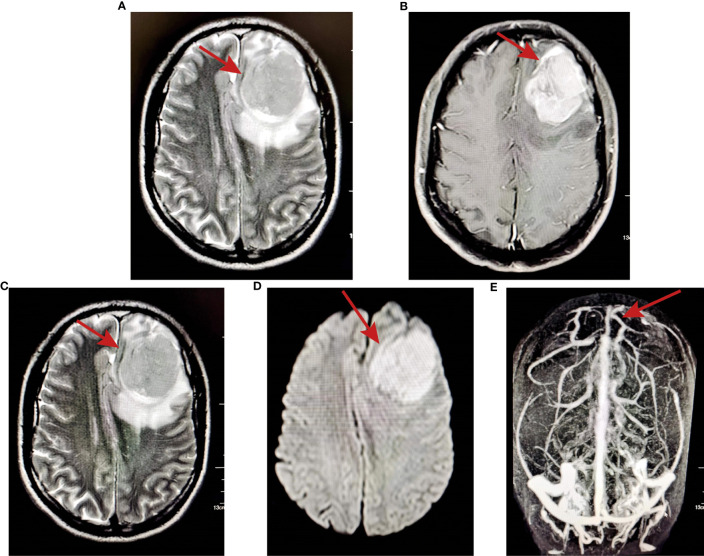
MRI examination showed a space-occupying lesion in the left frontotemporal lobe. (A) T2-weighted images; (B) T1-weighted images; (C) FLAIR showed slightly high signal and the boundary was still clear; (D) DWI showed high signal enhancement; (E) MRA showed that the compression of the bilateral anterior cerebral artery shifted to the right.
